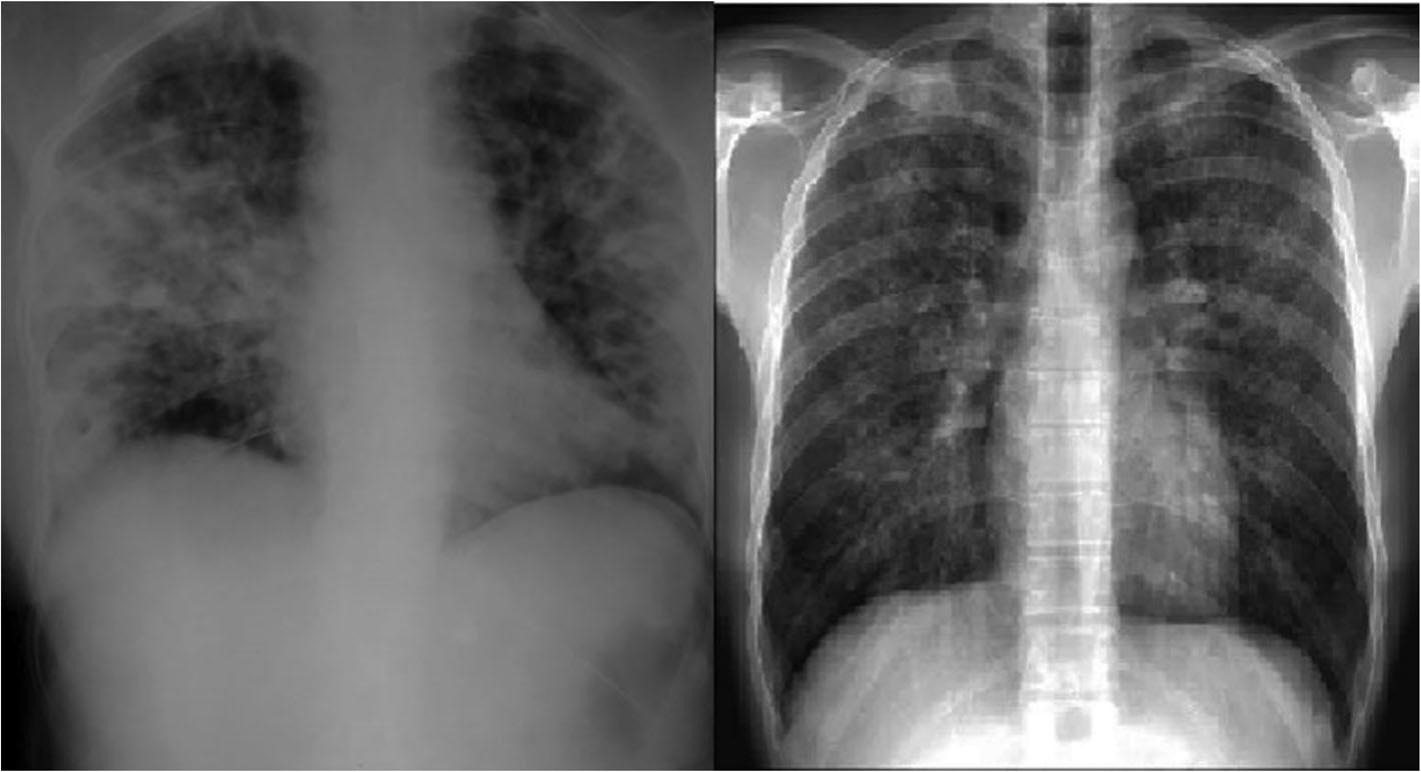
X-ray presentation of PCP positive patients showing multiple pulmonary involvement with massive pulmonary infiltrates
